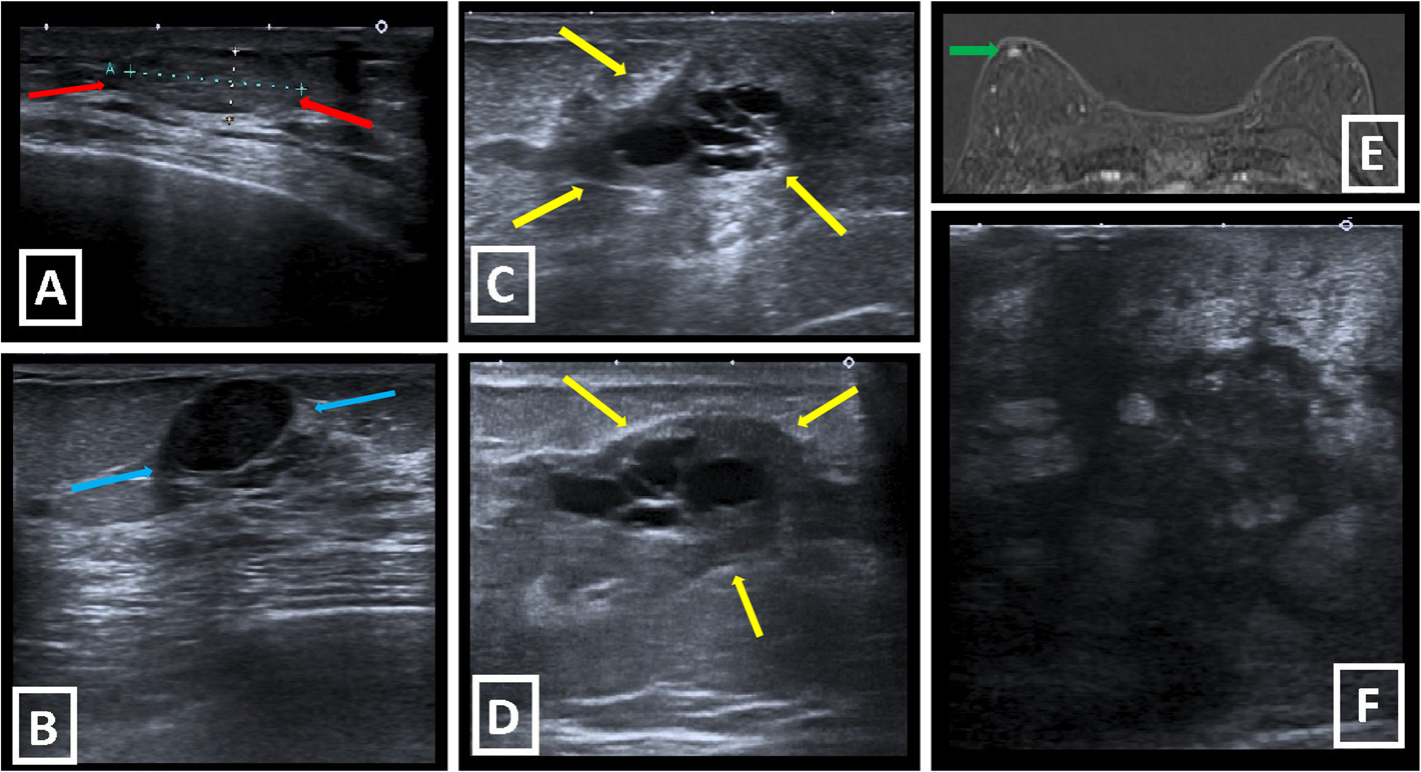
Uncommon male breast lesions due to fibroepithelial or lobular origin. a Fibroadenoma is seen as well-defined, hypoechoic, solid lesion with posterior acoustic enhancement in US (red arrows). b Circumscribed, hypoechoic, solid lesion with slight posterior acoustic shadowing is diagnosed as “Adenosis” after biopsy (blue arrows). c, d Fibrocystic changes with apocrine metaplasia. US shows multiseptated cystic structures within retroareolar heterogeneous hypoechoic area in a 59-year-old male patient with palpable mass (yellow arrows). e After detecting right retroareolar ductal ectasia with peripheral hypoechoic heterogeneous area in US (not shown), subtraction image of dynamic MRI of 72-year-old male patient shows retroareolar well-defined enhancing focus which corresponds to the defined lesion. Biopsy reveals the diagnosis of “Fibroadenomatoid changes”. f Large, heterogeneous, ill-defined, hypoechoic mass with subcutaneous edema and pectoral muscle invasion (not shown) is diagnosed as ILC
